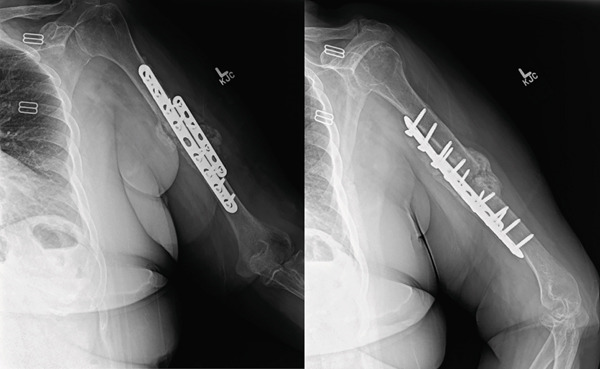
Radiographs taken 2 months postoperatively demonstrate acceptable alignment and improved callus formation from prior radiographs. Union of the fracture appears to be achieved.
